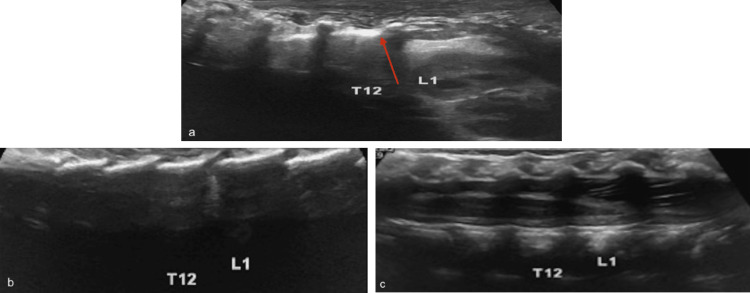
Rib shadow Lateral (a), paramedian (b), and midline (c) longitudinal ultrasound images demonstrating the last rib shadow (red arrow) on the T12 vertebra. Moving the probe medially while following the T12 vertebral shadow helps in assigning the levels of T12 and L1 vertebrae Radiological images obtained by the authors
